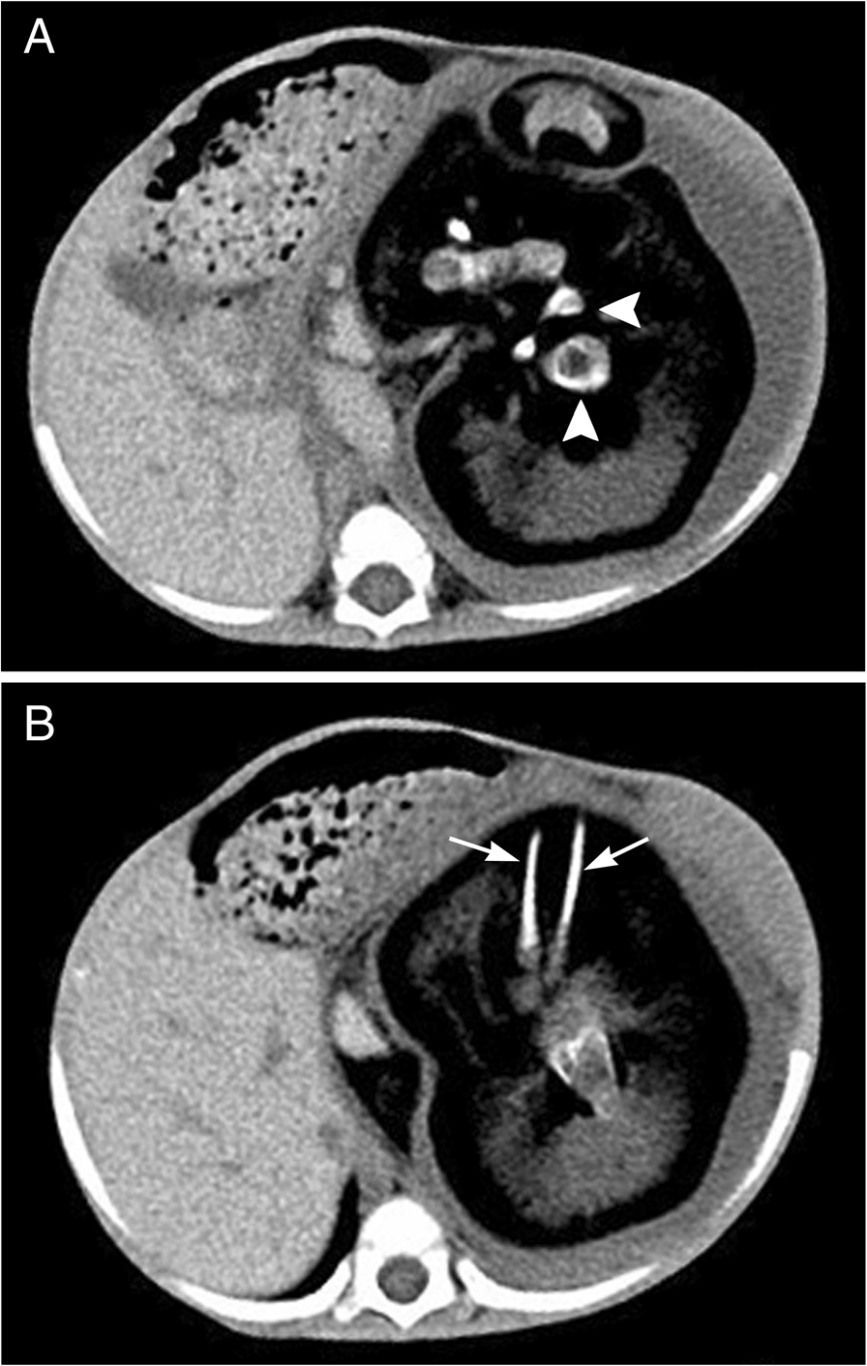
Abdominal computed tomography shows a semisolid mass with calcified and lipomatous components within the cyst. A. A well-formed vertebral body (Arrowhead); B. Arrow head shows the long opacities corresponding to fetal limbs.
